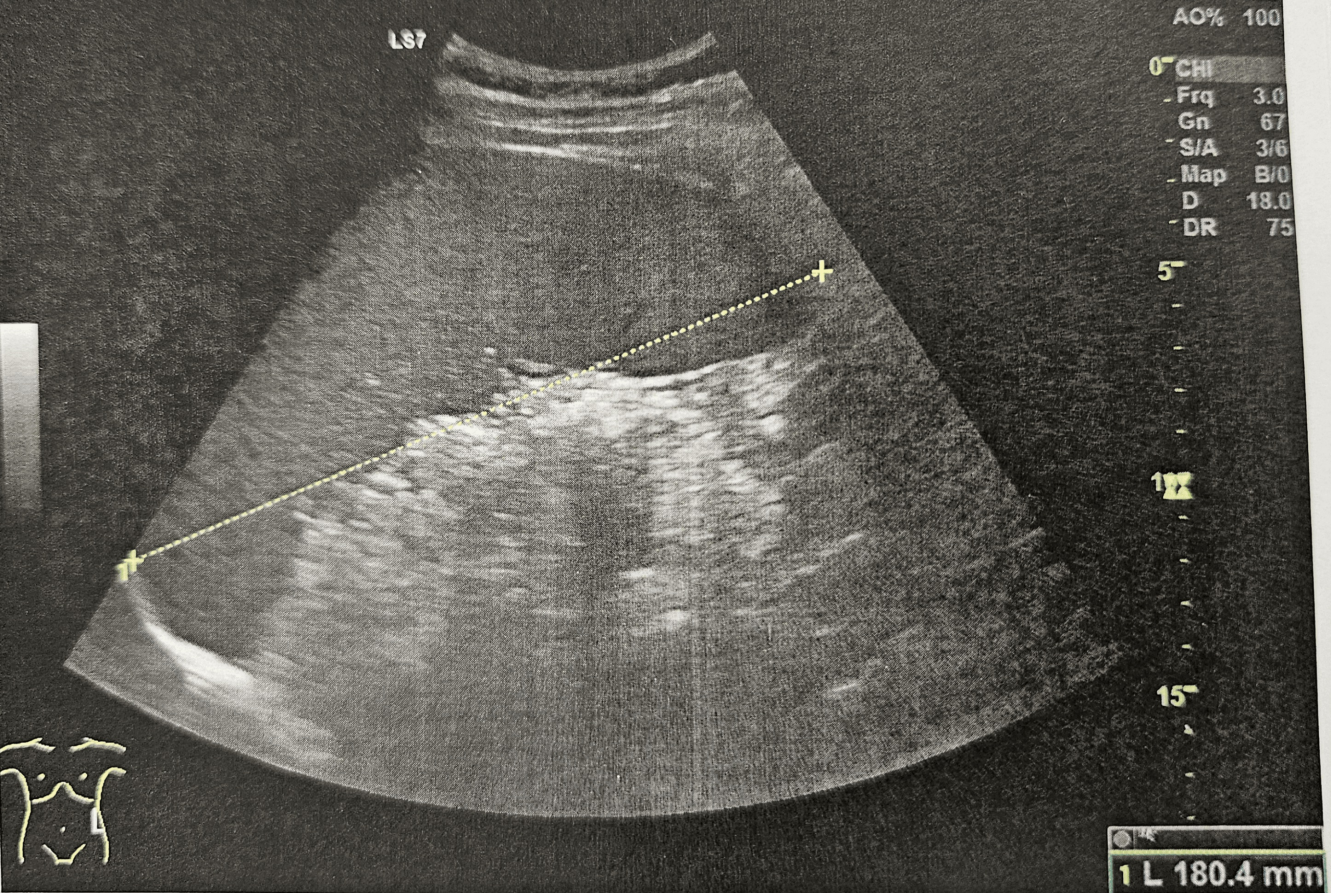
Ultrasound image of splenomegaly.
